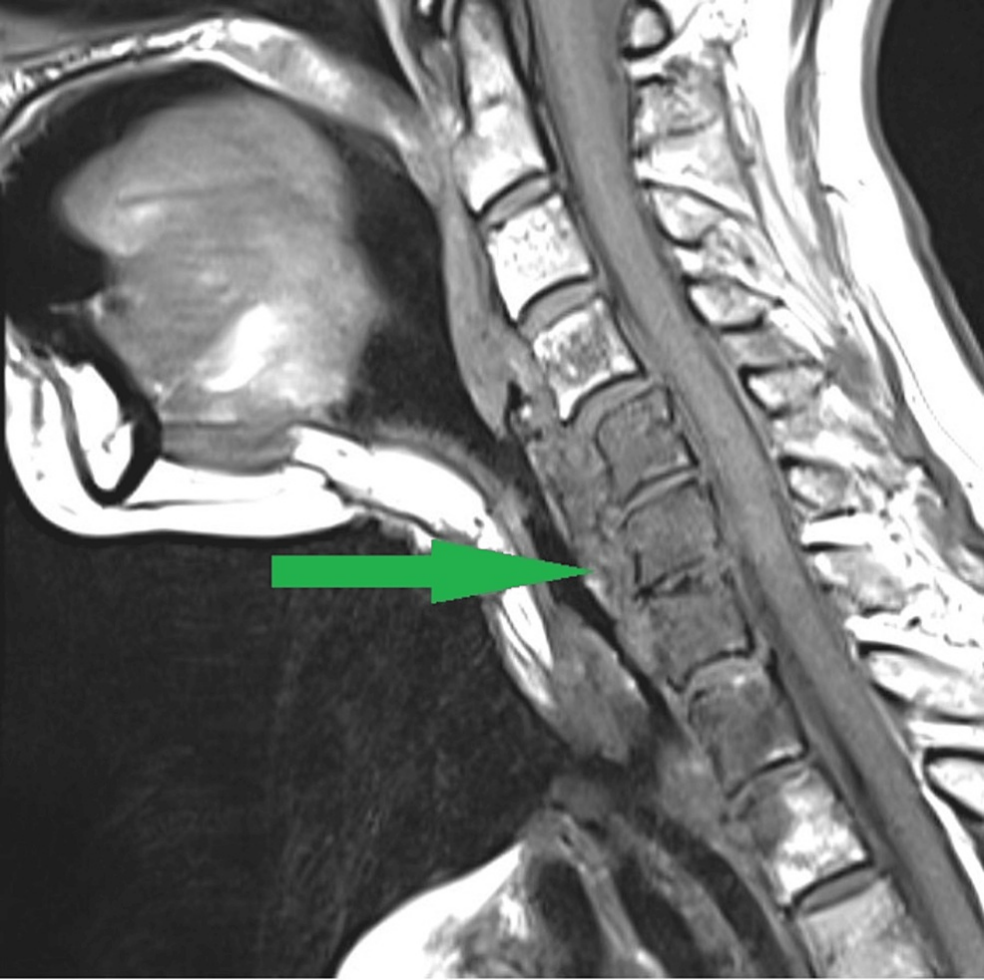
Magnetic resonance imaging of the cervical spine demonstrating an abscess in the retropharyngeal space
